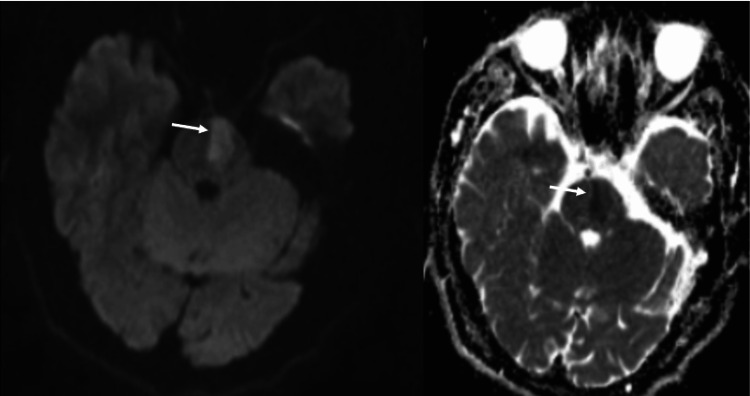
Local county hospital cranial MRI shows left pontine infarction (arrow indicated)
